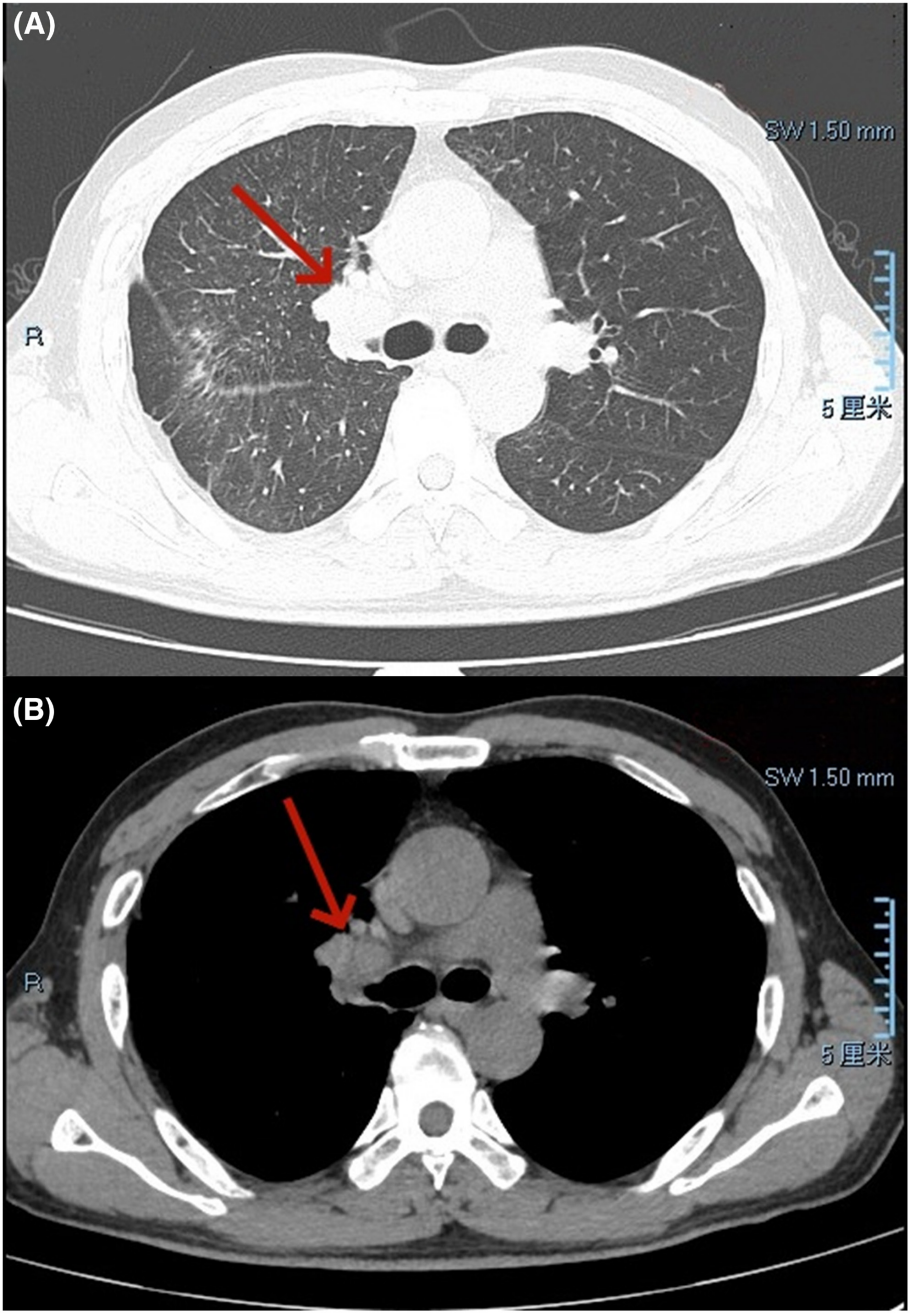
Chest computed tomography (CT) showed a mass occupying the right hilus, with unclear boundaries, size 4.6 × 3.6 cm. Tumor recurrence was diagnosed. (A) Represents the lung window manifestation on chest CT plain scan; and (B) Represents the mediastinal window manifestation.
